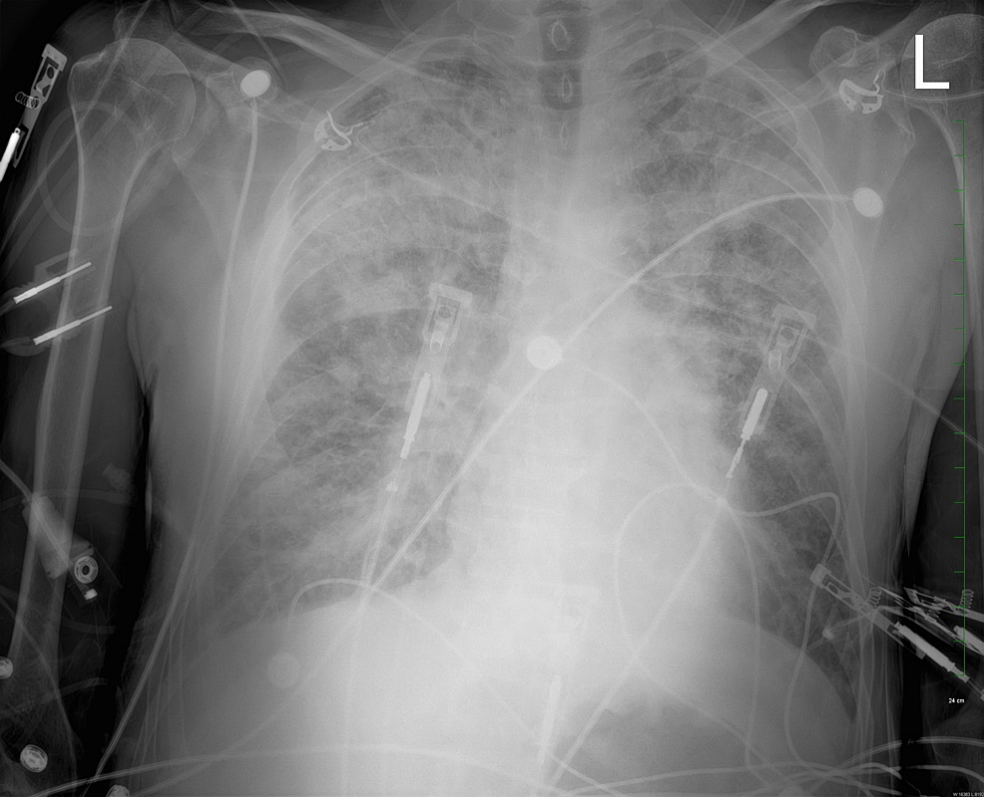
Chest X-ray reveals marked exacerbation of airspace opacification bilaterally, notably in the upper lobes during the ninth day of hospitalization.
